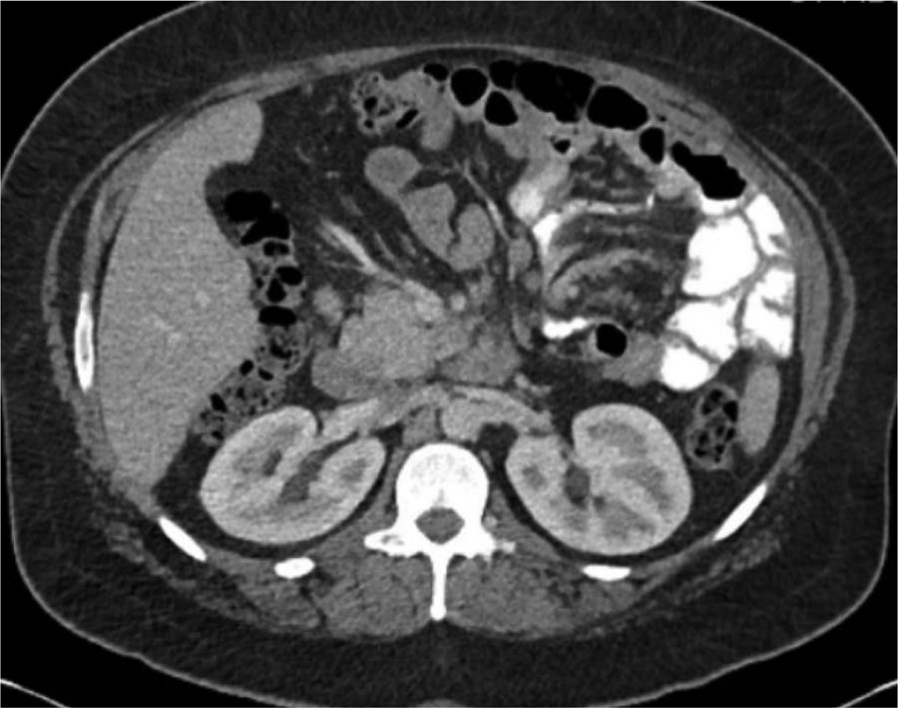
Axial CT demonstrating mesenteric swirling.
